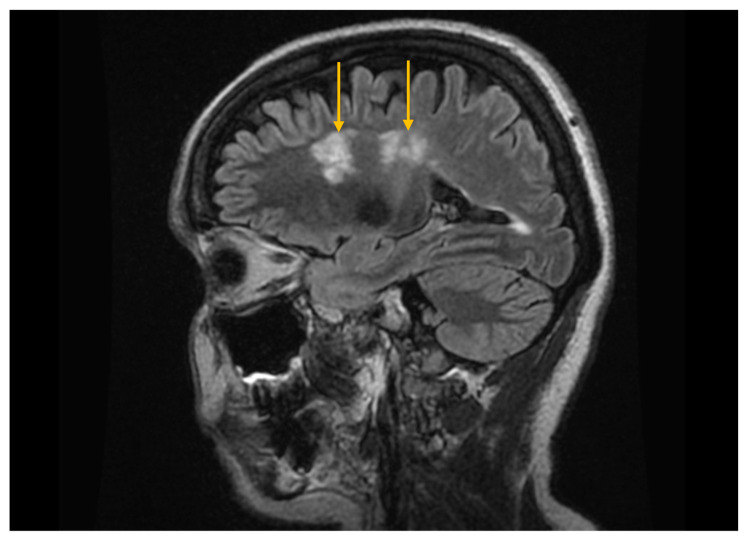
Magnetic resonance imaging (MRI) of the brain using the fluid-attenuated inversion recovery (FLAIR) sequence. Sagittal FLAIR MRI image demonstrates hyperintense cerebral demyelinating lesions (arrows), with a distribution pattern similar to that seen in multiple sclerosis.
